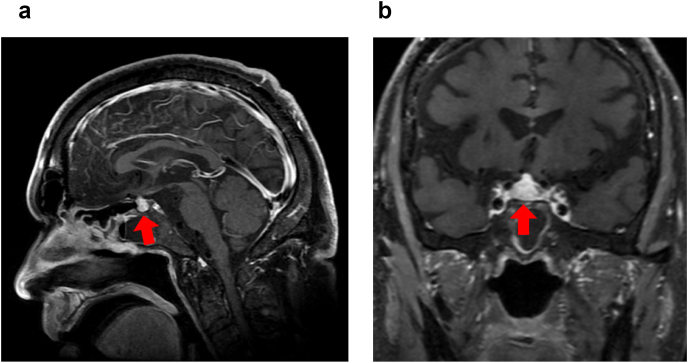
Magnetic resonance imaging reveals pituitary enlargement.
